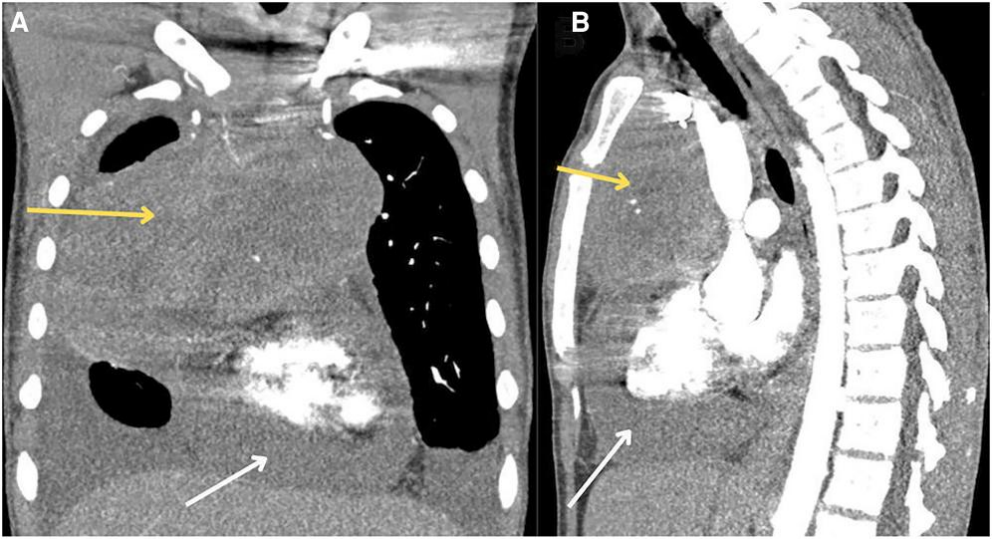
(*A*) Computed tomography chest coronal view demonstrating large anterior mass (yellow arrow), large pericardial effusion (white arrow), and bilateral pleural effusions. (*B*) Computed tomography chest sagittal view demonstrating large anterior mass (yellow arrow) and large pericardial effusion (white arrow).
